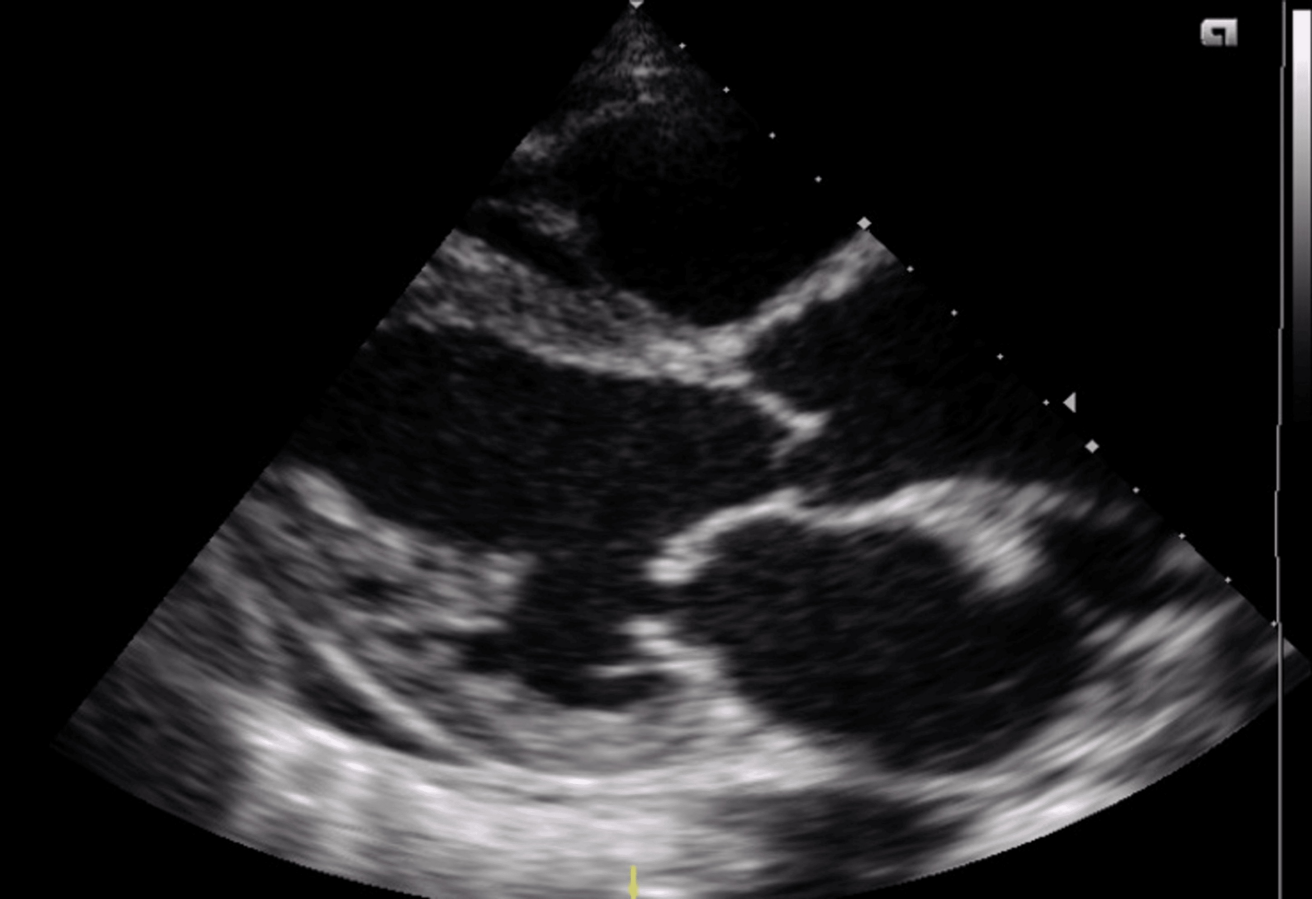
Parasternal long axis of the heart showing dilated chambers
